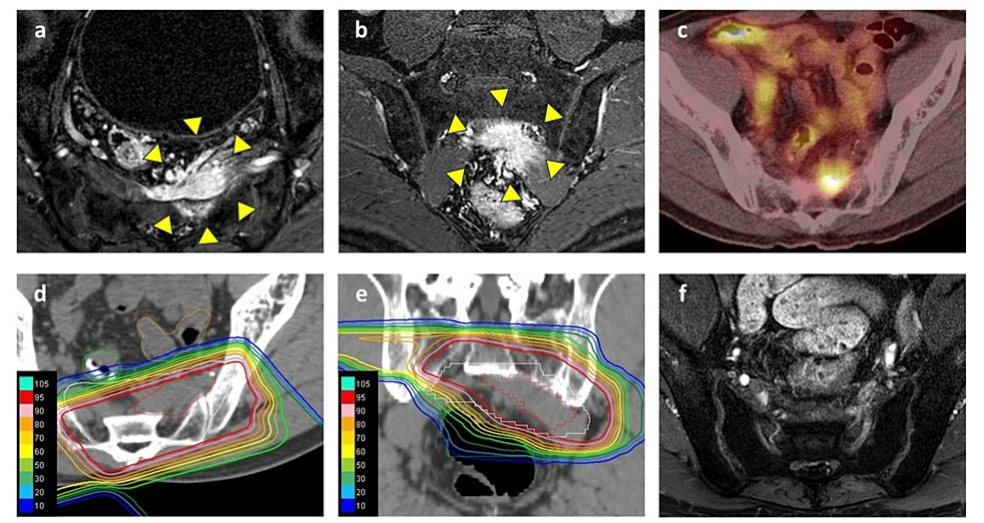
MRI and FDG PET-CT images pre and post-first PBT for presacral recurrence Contrast-enhanced MRI (a: axial, b: coronal), FDG PET-CT (c: axial), and dose distribution of first PBT (d: axial, e: coronal). Post-treatment MRI (f: axial) showed a complete response one year after the first PBT. In the images, the recurrent lesion is indicated by a yellow triangle. The dose lines are color-coded as follows: Red line: 95% isodose line, Yellow line: 75% isodose line, Green line: 50% isodose line, Light blue line: 25% isodose line. FDG PET-CT: 18 F-fluorodeoxyglucose Positron Emission Tomography–Computed Tomography; PBT: Proton Beam Therapy.
